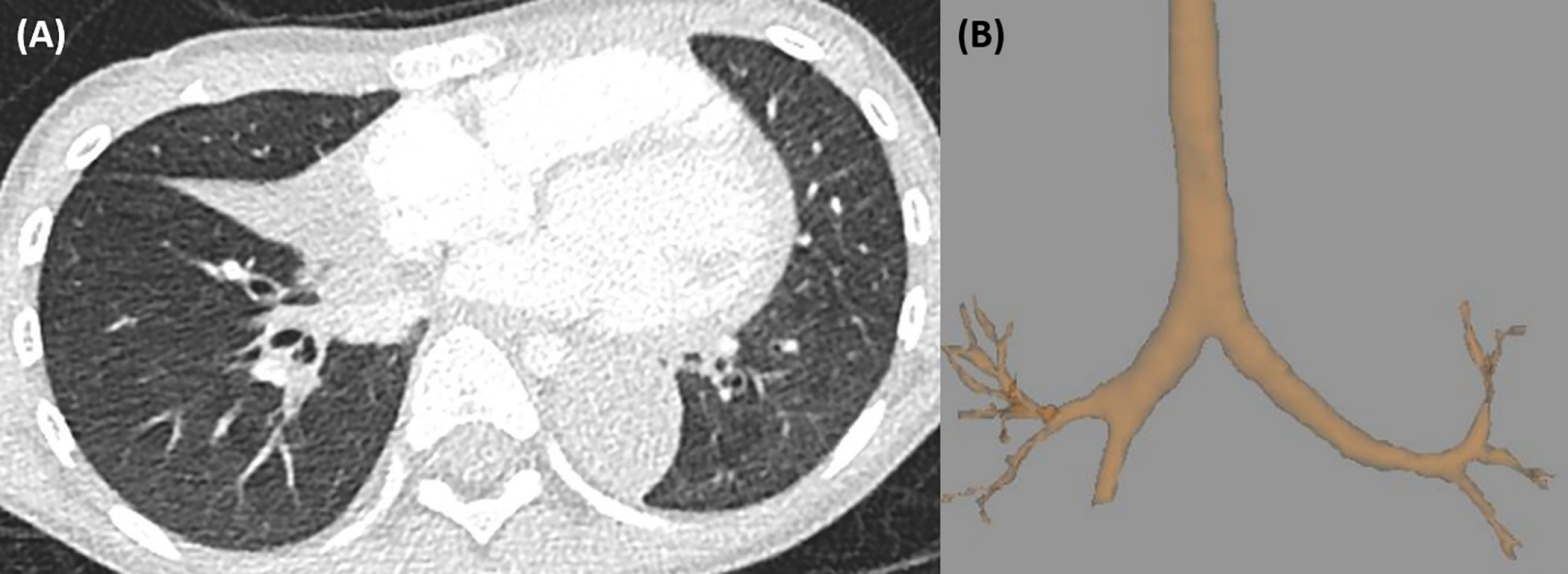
A representative case showing failure of an attempted quantitative airway analysis of a four-year-old boy with post-infectious bronchiolitis obliterans. (A) An axial CT image shows atelectasis of right middle lobe and left lower lobe. Airways are not delineable in the affected areas. (B) Airway segmentation failed in the corresponding and distal regions.
